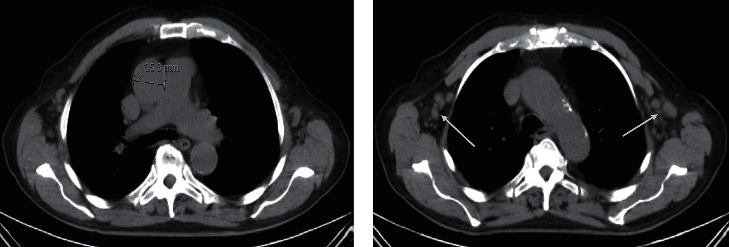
(a) CT scan showing mediastinal lymphadenopathy measuring 35.3 mm (marker); (b) CT scan showing bilateral axillary lymphadenopathies (arrows).
